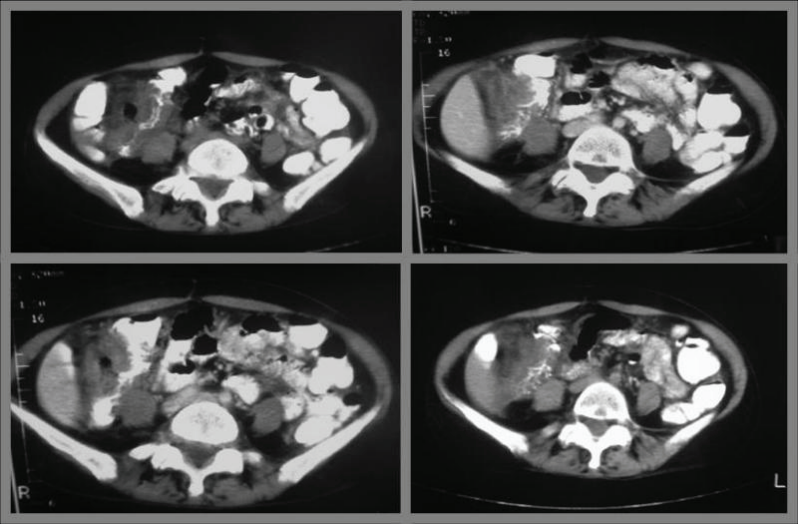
Non-contrast (Top left) and contrast (Top right, Bottom left, Bottom right) enhanced abdominal CT scan shows a segment of narrowing with shoulder formation of ascending colon and minimal inflammatory changes of adjacent fat.
